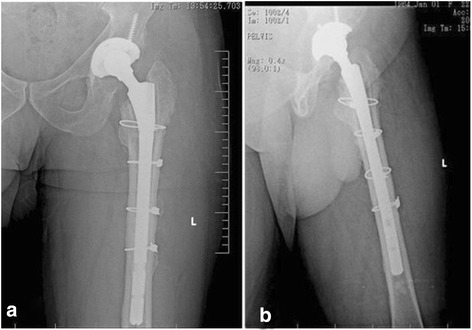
a Anteroposterior radiograph. b Lateral radiograph of a revised cemented femoral stem demonstrates good fill and fit of the cementless modular stem. The trochanteric osteotomy flap was fixed carefully, and the osteotomy site was consolidated
